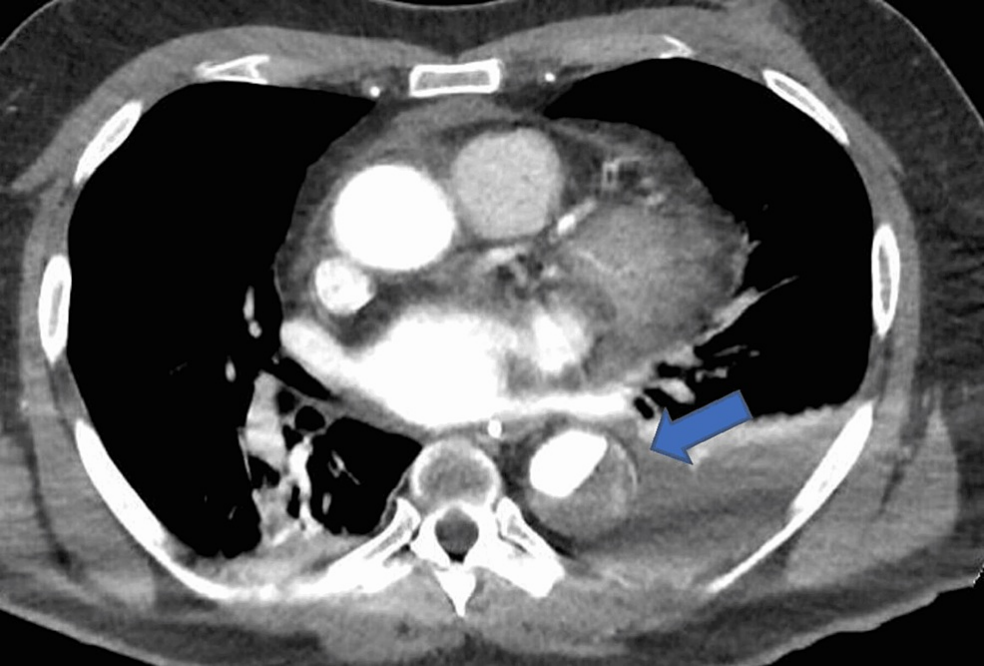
Computed tomography aortogram scan showing acute type B aortic dissection as shown by the pointed arrow
